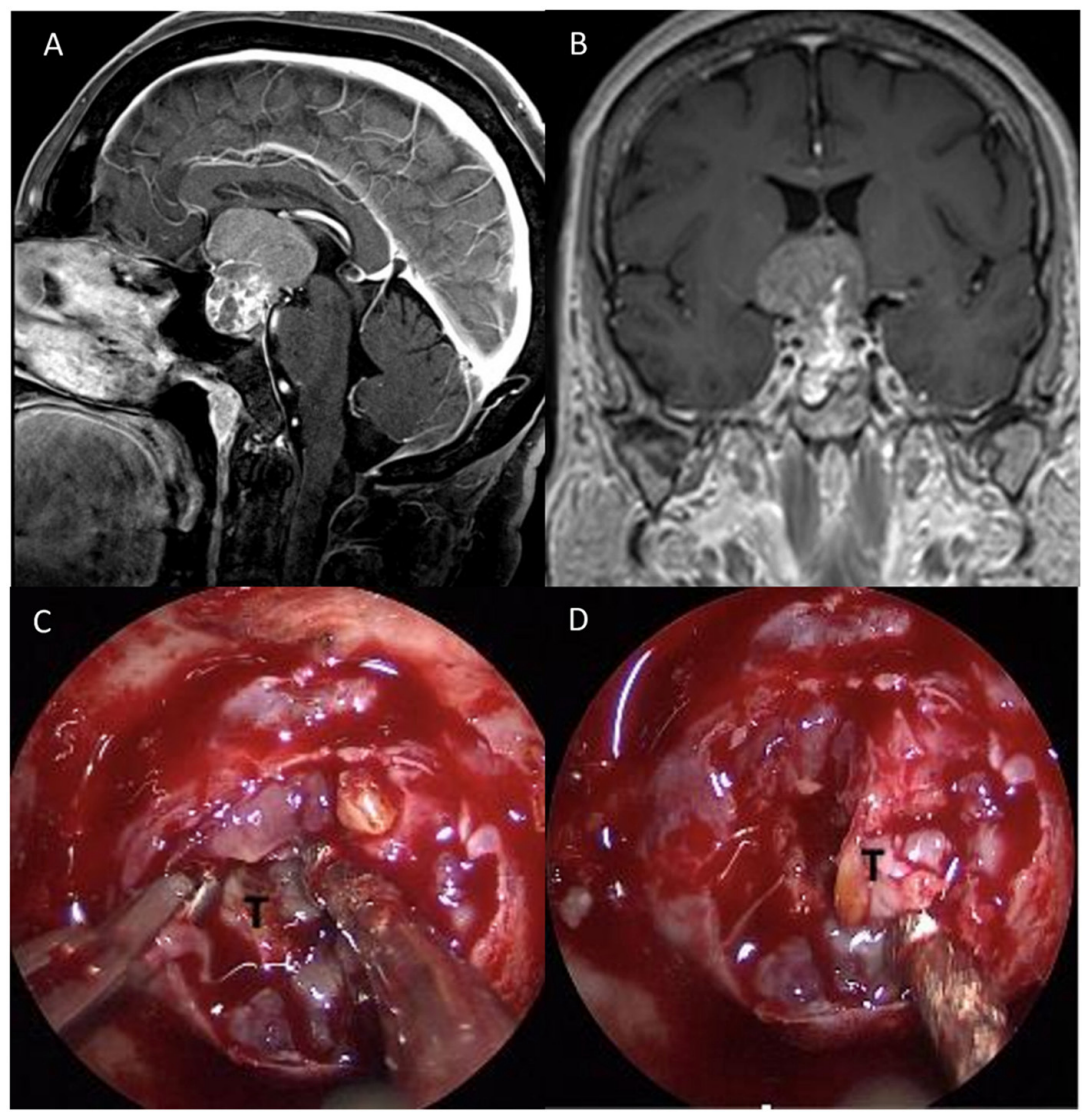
Preoperative sagittal (A) and coronal (B), post-gadolinium MRI scan showing a suprasellar mass (Dmax 3.9 cm) with the third ventricle involvement and heterogenous contrast enhancement compressing the optic chiasm. (C) After dural opening, (D) a fibro-elastic and infiltrating lesion was partially removed in order to obtain optic nerve decompression.
